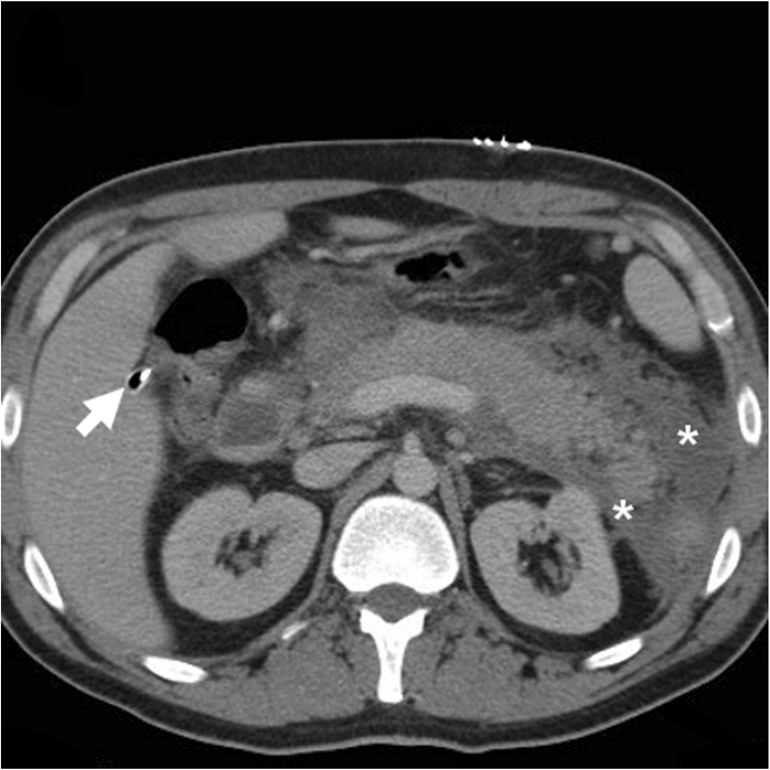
Postoperative acute pancreatitis (serum lipase 15,000 U/L) after laparo-endoscopic intraoperative rendezvous cholecystectomy. Twenty-four hours after surgery, contrast-enhanced CT with drainage in place (*thick arrow*) showed preserved enhancement of the pancreatic gland and development of peripancreatic effusion (*). The patient was treated with positioning of nasobiliary drain and eventually developed pseudocyst formation
